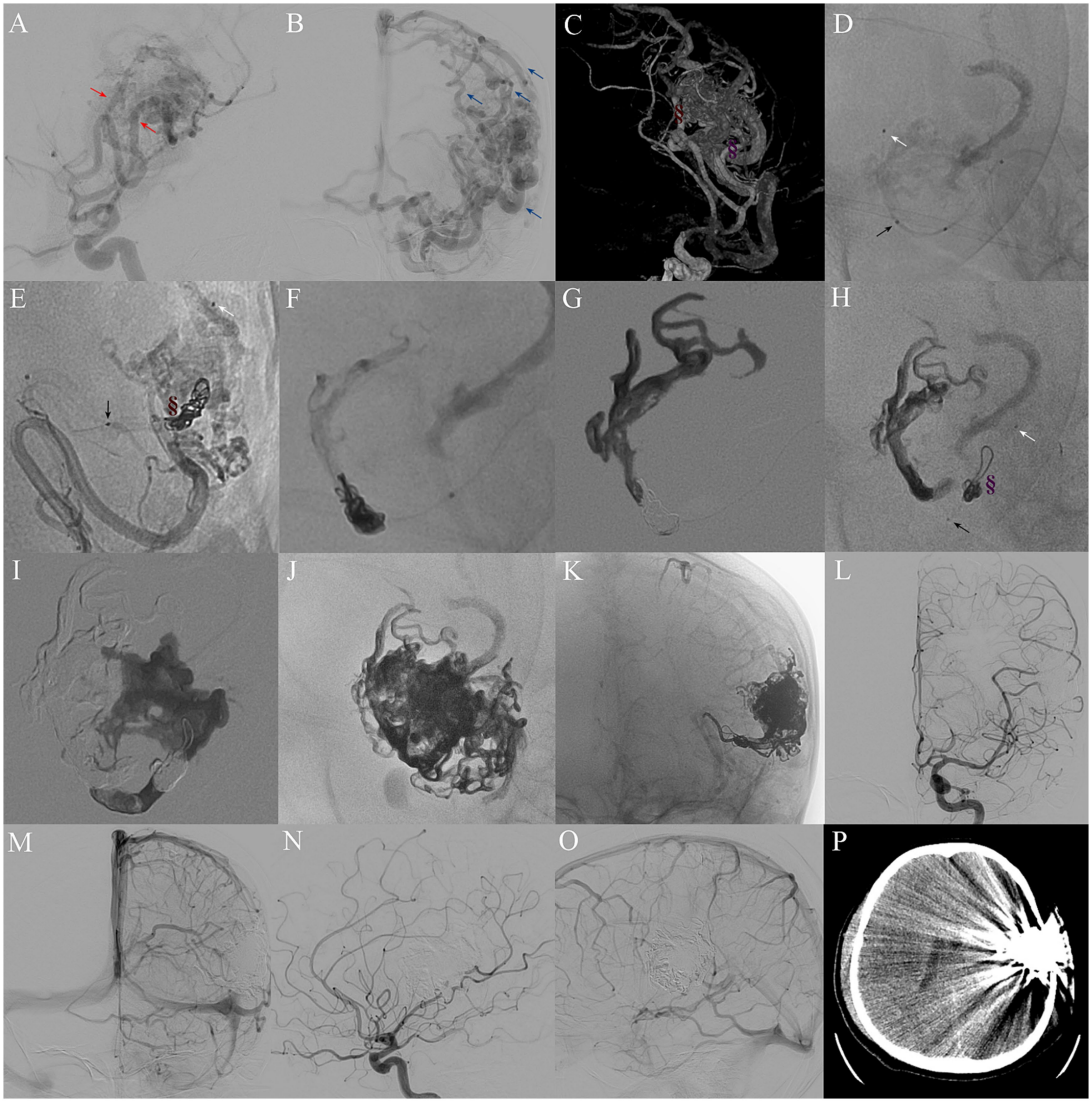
Procedure of mPCT embolization for complete nidus occlusion. (A) Preoperative DSA indicating a 5.2 cm bAVM nidus, supplied by two main feeders from the left middle cerebral artery (red arrows) and one feeder from the left anterior cerebral artery, and (B) drained by cortical venous to the superior sagittal sinus and also drained downwards into the basilic vein (blue arrows). (C) Location of two plugs comprising coils and Onyx in feeders (brown and purple symbols). (D,E,H) Single shot fluoroscopy showing the markers of two microcatheter tips: Apollo (white arrow) and Wissky (black arrow) in the same selective feeder. (E–G) Formation of a coil-Onyx plug (brown symbol) to control reflux. (H,I) Selection of another feeder for a second mPCT embolization. (J,K) Post-embolization DSA revealing Onyx cast. (L–O) Immediate postoperative selective left ICA DSA confirming complete nidus exclusion. (P) Postoperative brain CT showing no hemorrhage or infarction.
